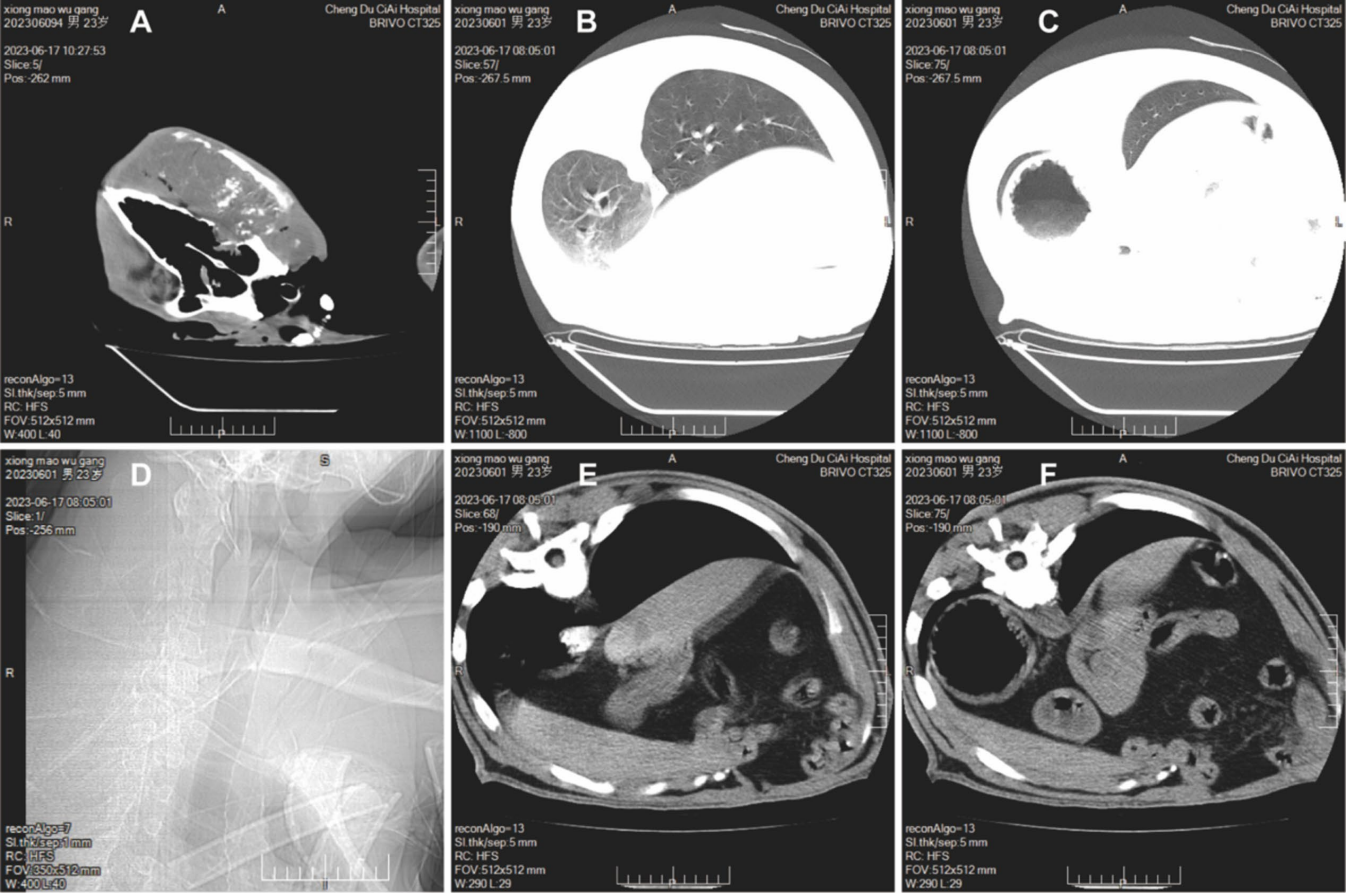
CT scans of giant panda Wugang. (A) A mass is visible in the right maxillofacial region. (B) A bulla is observed in the left lower lobe of the lung. (C) Pleural effusion is present. (D) Degenerative changes in the vertebrae. (E) Enlargement of the left adrenal gland with multiple calcified foci. (F) Swelling of the left psoas major muscle with a visible mass.
